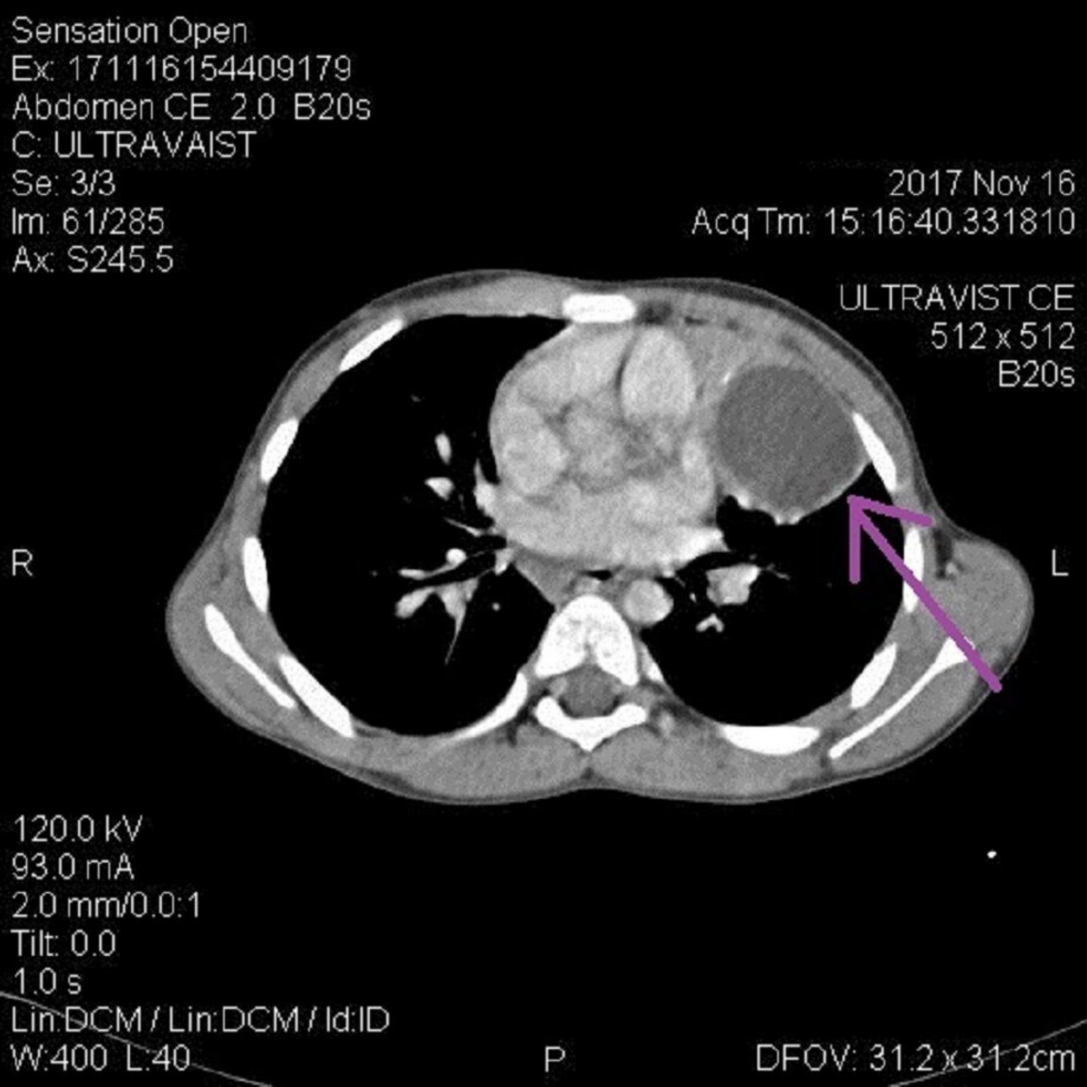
Computed tomography (CT) findings Arrow showing left lower lung lobe cyst.
